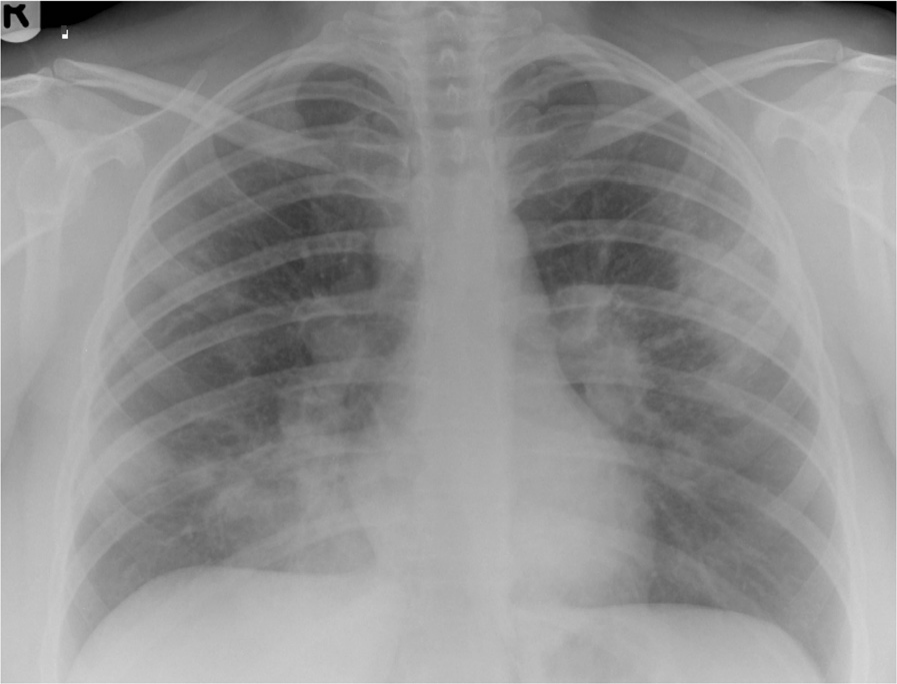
Plain chest radiograph demonstrating bilateral patchy air-space consolidation and prominent hilar shadows.
